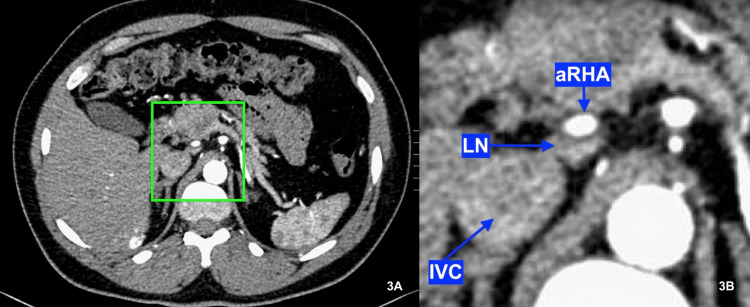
CT scan image showing the relationship between the aRHA and the related lymph nodes. (A) Axial CT image showing the aRHA arising from the SMA. (B) Magnified image of axial CT showing the main trunk of the aRHA covered posteriorly by the LN. IVC: Inferior vena cava; aRHA: aberrant right hepatic artery; LN: lymph node; CT: computed tomography
